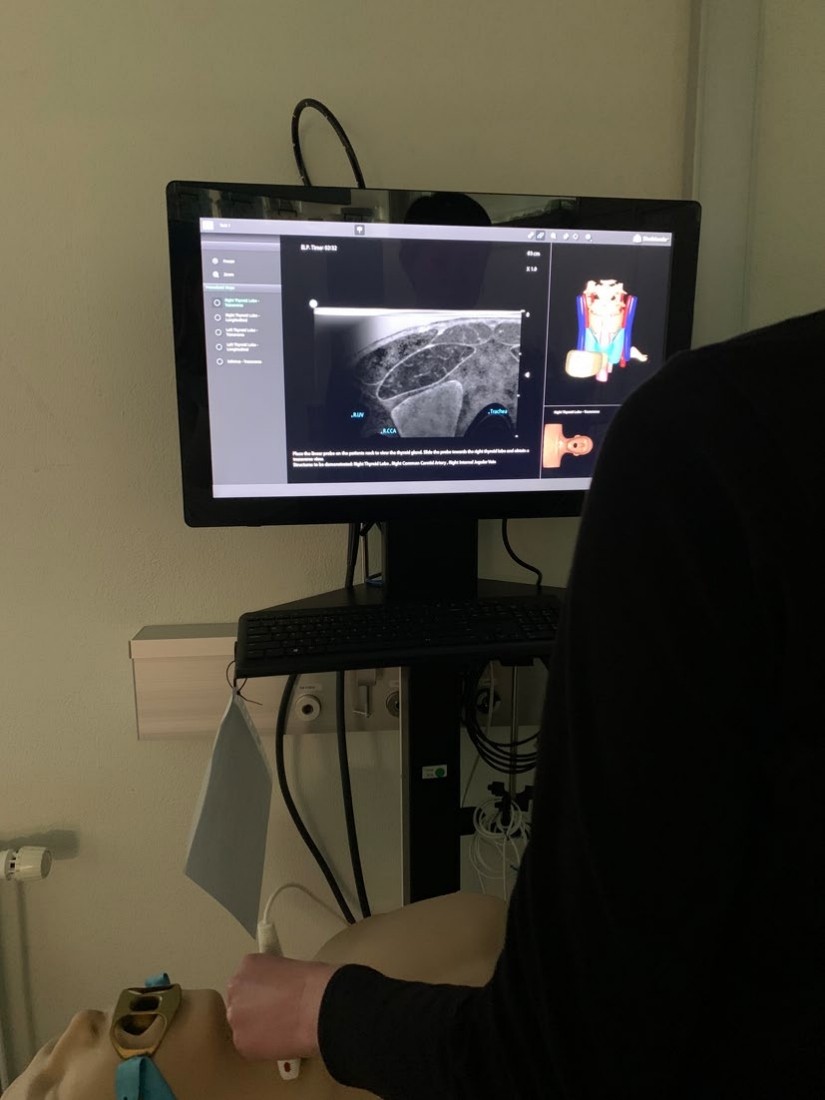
Picture of Task 1 in the neck ultrasound module of the U/S mentor
